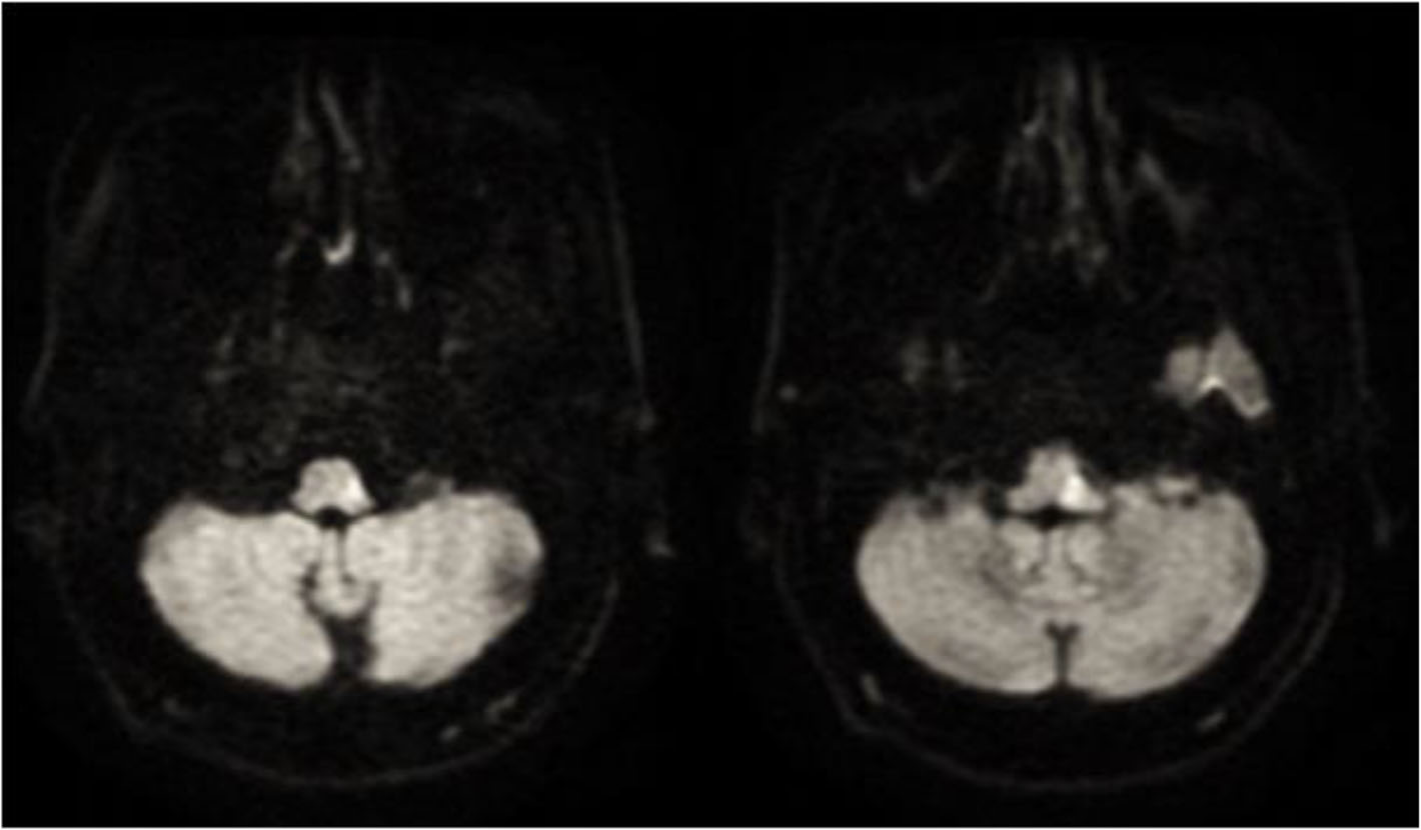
Left lateral medullary infarction along axial magnetic resonance imaging
